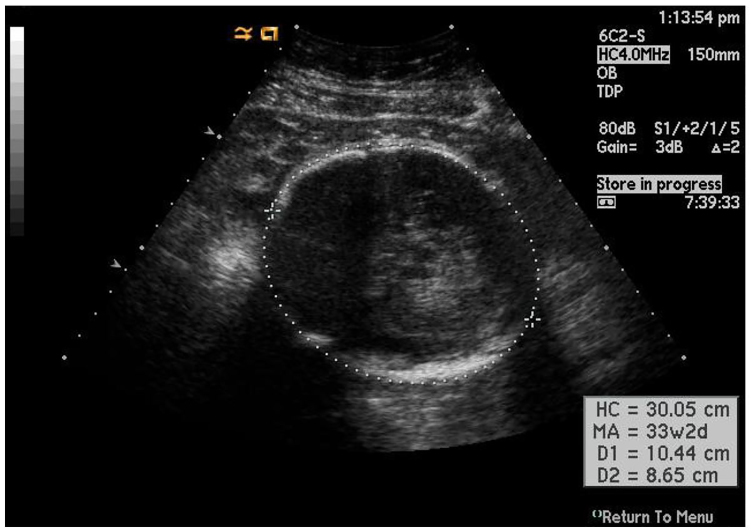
Obstetric ultrasound image depicting normal fetal anatomy at a gestational age of approximately 33 weeks and 2 days, with head circumference of 30.05 cm (Image courtesy of http://www.learnobultrasound.com/datinggrowth.htm).
